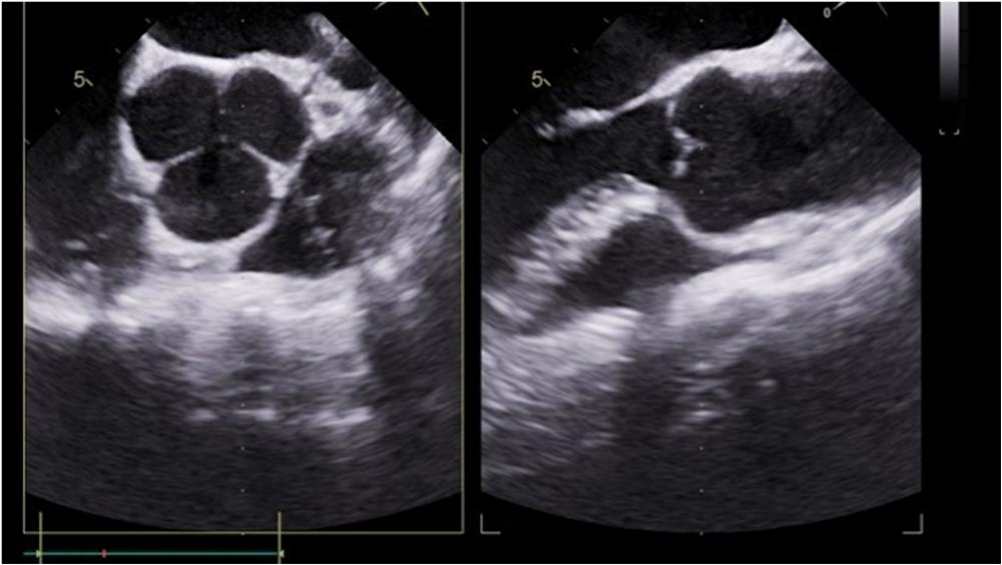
Transoesophageal echocardiography: normal looking aortic valve after antithrombotic treatment.
